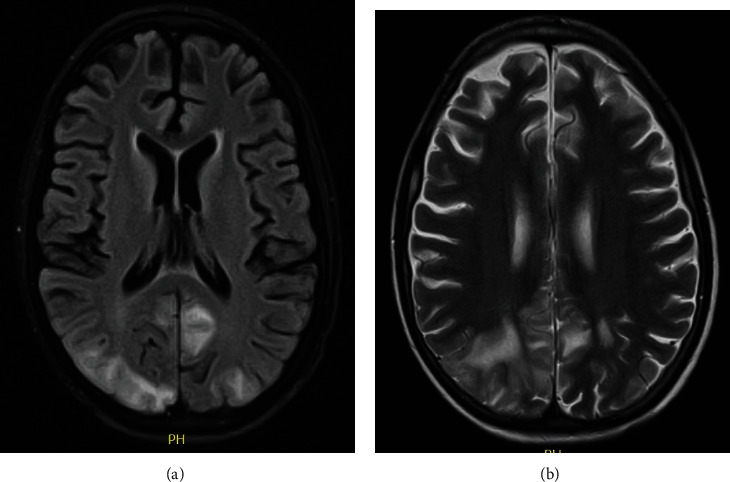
Brain MR of case 3. (a) Fluid-attenuated inversion recovery sequence showing occipitoparietal hyperintensities and (b) its representation in T2-weighted sequence.
